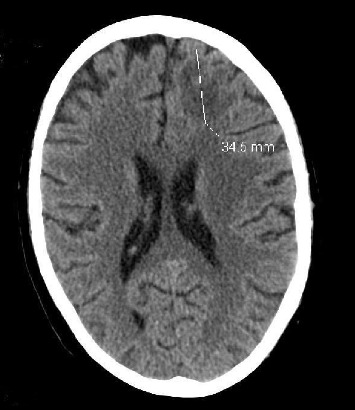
Axial CT head without contrast showing low attenuation involving predominantly the white matter of the left frontal lobe shown with measurements. No shift of the midline structures was noted.
